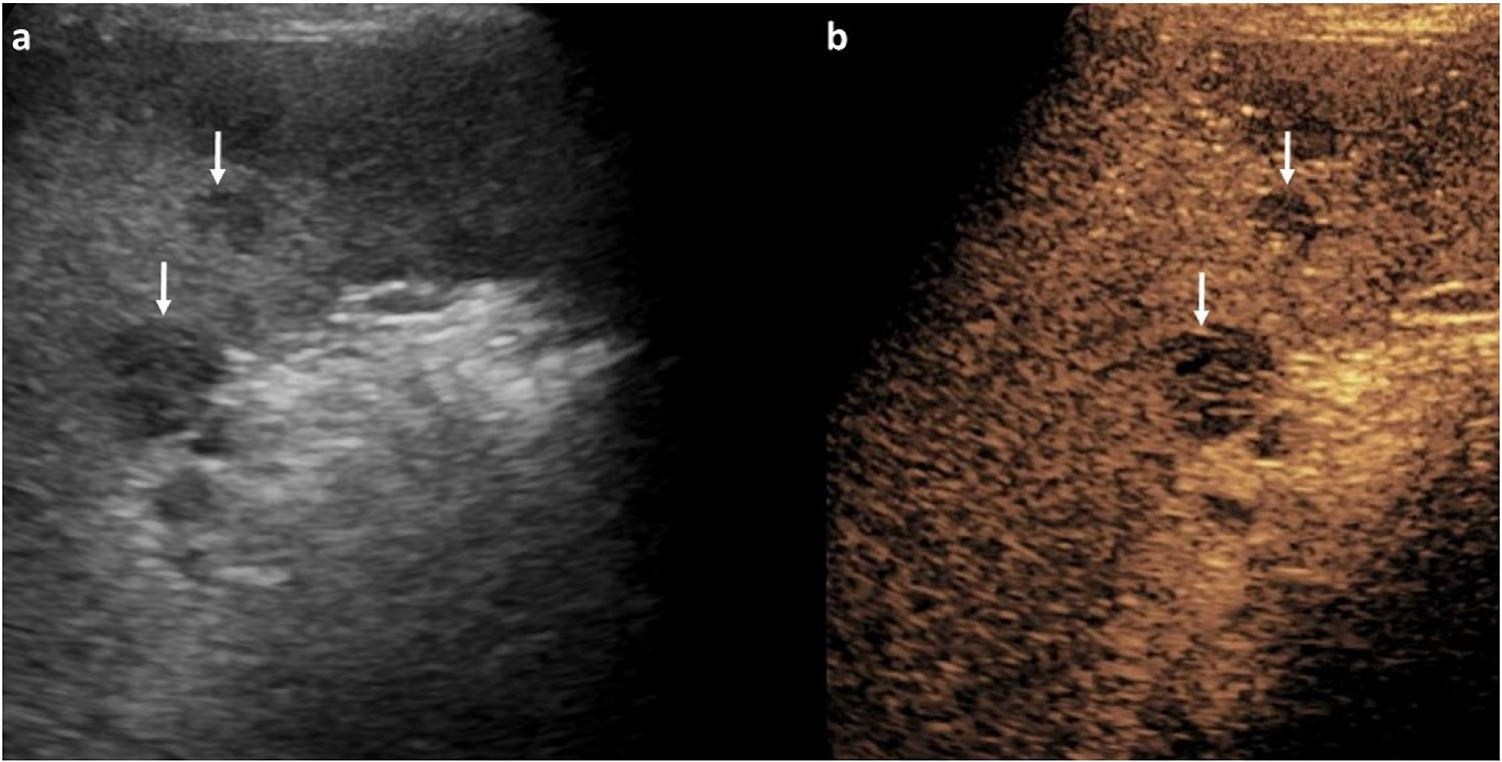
18-year-old male. Contrast ultrasound of the spleen in a patient with multiple splenic lesions, undergoing chemotherapy showing multiple hypoechoic lesions on a grayscale with the differential including malignant deposits or fungal dissemination; these were all hypoenhancing following b contrast administration, in keeping with disseminated fungal disease
